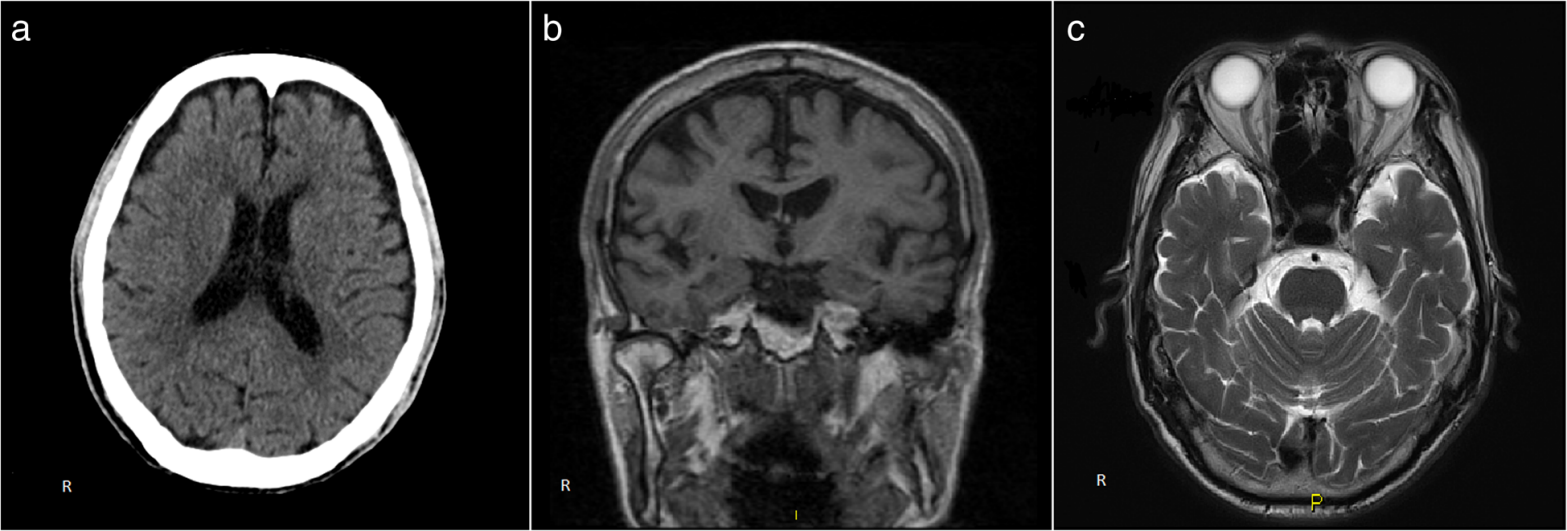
a Axial CT brain of our patient showed atrophy of bilateral frontal lobes (b) T1 weighted coronal MRI brain showed preservation of the hippocampi (c) T2 weighted axial MRI brain showed preservation of bilateral medial temporal lobes
